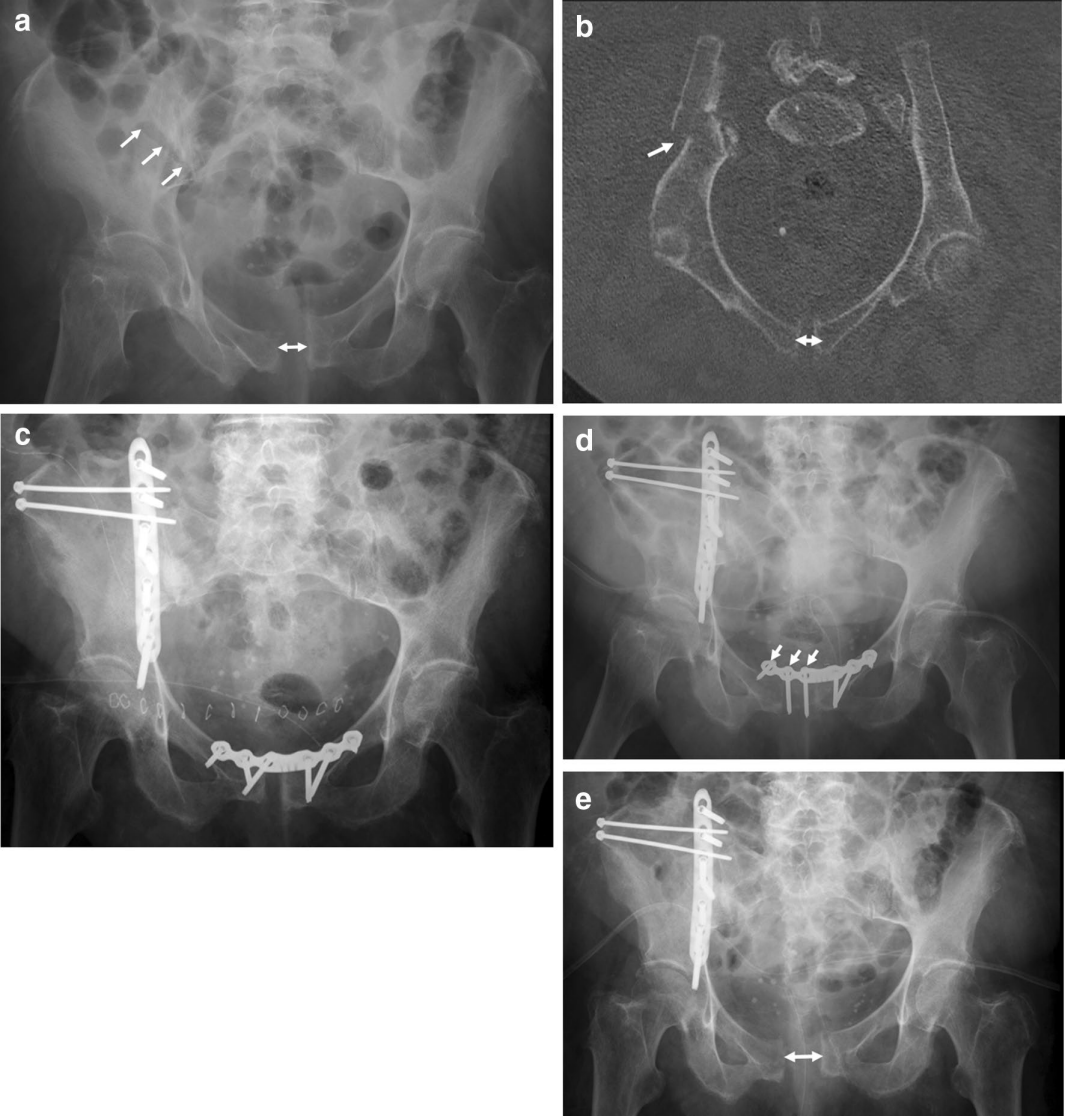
A 92-year-old female suffered a fall at home. The pelvic a.-p. overview shows a diastasis of the pubic symphysis and a fracture line at the right ilium (arrows) (a). CT-reconstruction along the pelvic brim shows the fracture of the right ilium and the diastasis of the pubic symphysis (arrows). The patient has a FFP type IIIa (b). Postoperative a.-p. pelvic overview. The ilium fracture and the pubic diastasis have been treated with open reduction and plate and screw osteosynthesis (c). Pelvic a.-p. overview two weeks after surgery. The three right screws of the pubic plate osteosynthesis show loosening. There are signs of surgical site infection. The symphysis plate needs to be removed and serial debridement becomes necessary (d). A.-p. pelvic overview after one month. The pubic diastasis has recurred. Due to surgical site infection at the ilium, serial debridement of the wound at the ilium is also needed (e)
